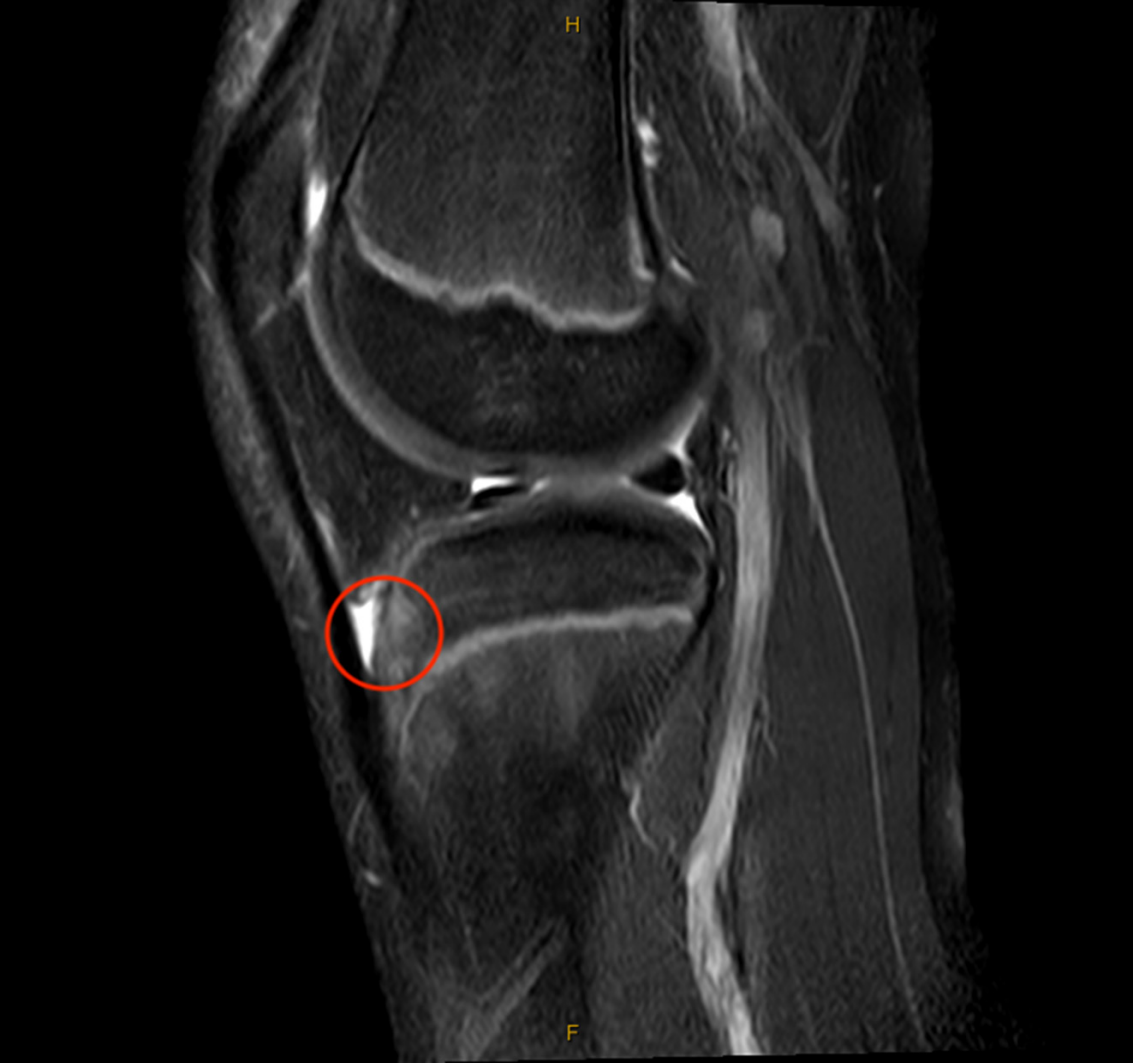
Sagittal MRI; Proton density fat‐saturation sagittal MRI sequence of the left knee obtained 8 days after the initial injury showing mild bone marrow edema in the tibial tubercle and a small amount of reactive fluid in the adjacent deep infrapatellar bursa (circle).
